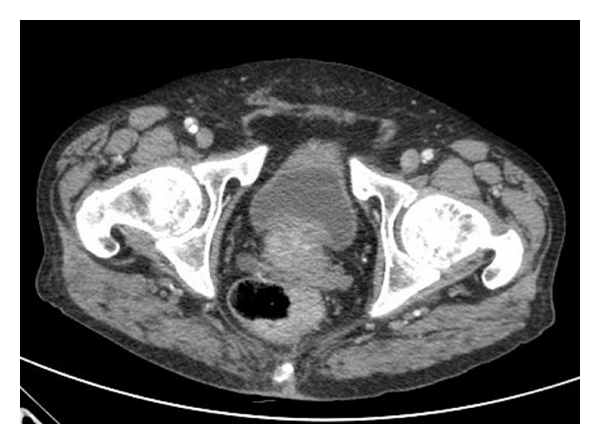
CT scans which show rectal mass.
